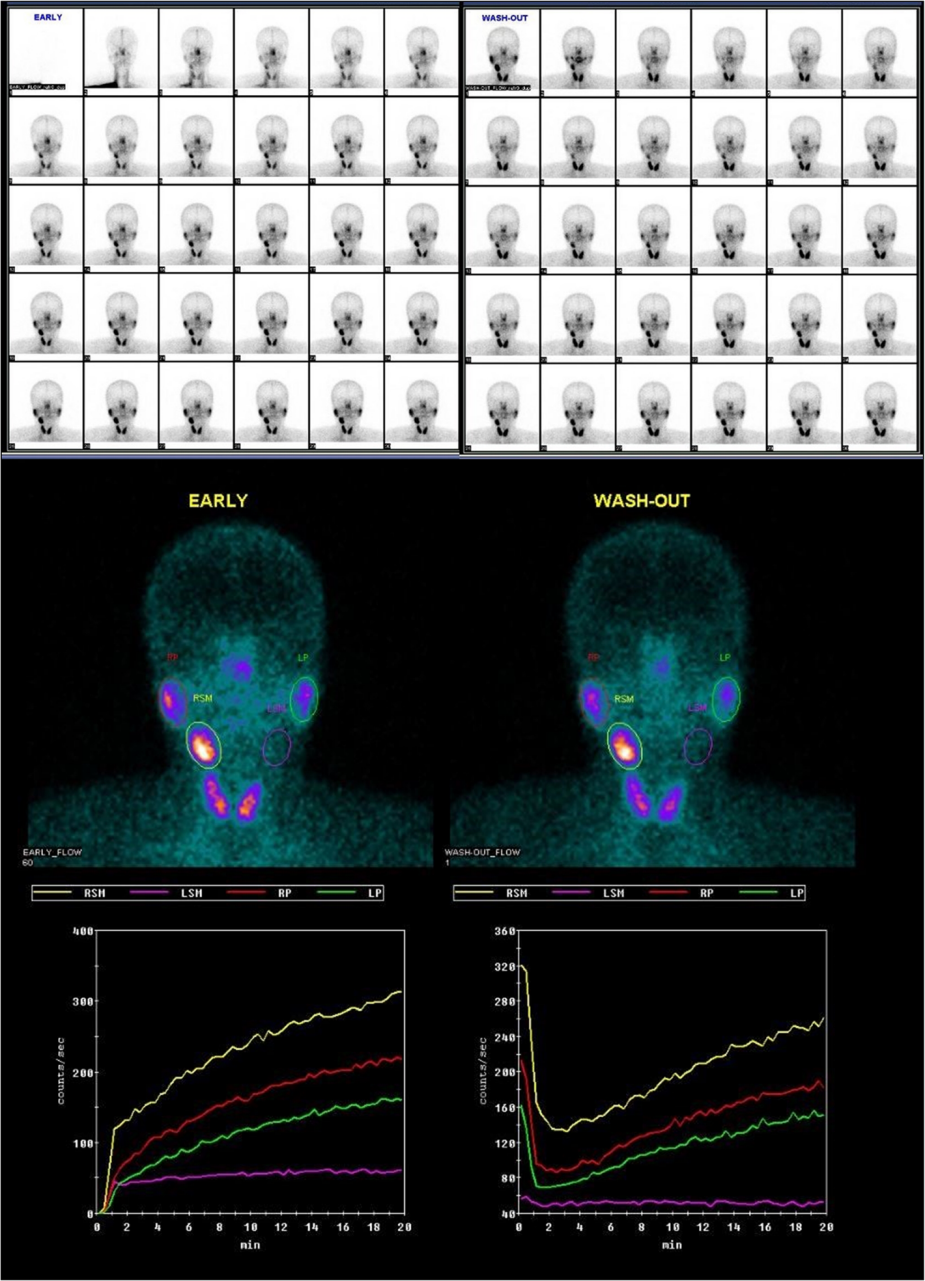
Preoperative technetium-99m pertechnetate salivary gland scintigraphy revealed that right salivary glands were within normal limits but with no definite radiotracer excretion in the Lt. submandibular gland
